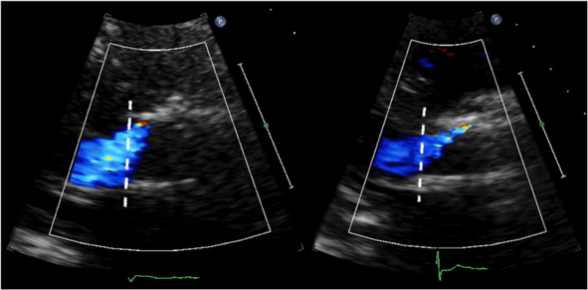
Two examples of eccentric PVL jets spreading out in all directions into the inflow area of the stent frame as seen in a parasternal long-axis view. Recording an image just below the stent frame (see dashed line) as recommended in the VARC publication will not show the “origin” or “neck” of the jet
